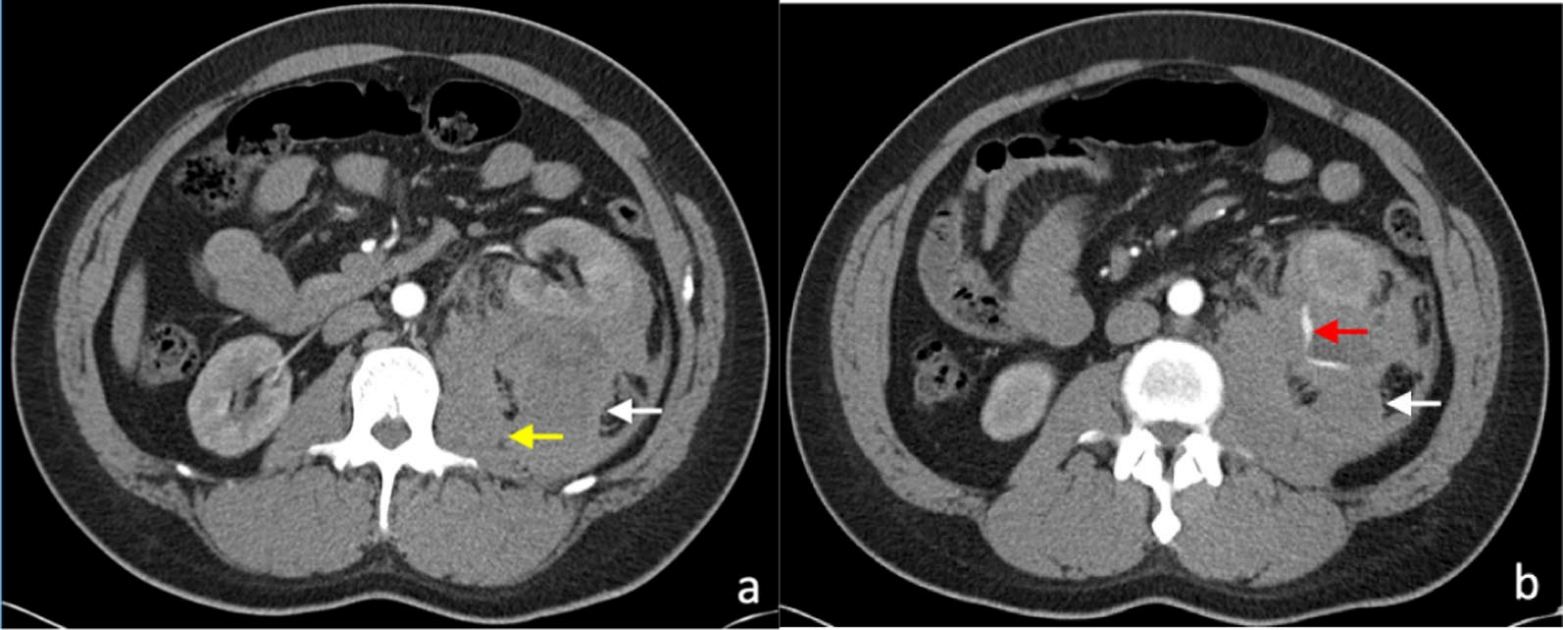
Post-contrast arterial phase axial CT images of a patient following two 14-gauge non-targeted core biopsies of the left kidney, demonstrating (a) left perinephric haematoma (white arrow). There is also a haematoma of the left psoas muscle, which is expanded (yellow arrow). b Active extravasation of contrast from a left inferior segmental renal artery (red arrow) was demonstrated inferiorly within the perinephric haematoma (white arrow). The active haemorrhage was successfully treated with embolisation coils of the left inferior segmental renal artery. The core biopsies confirmed IgA nephropathy
